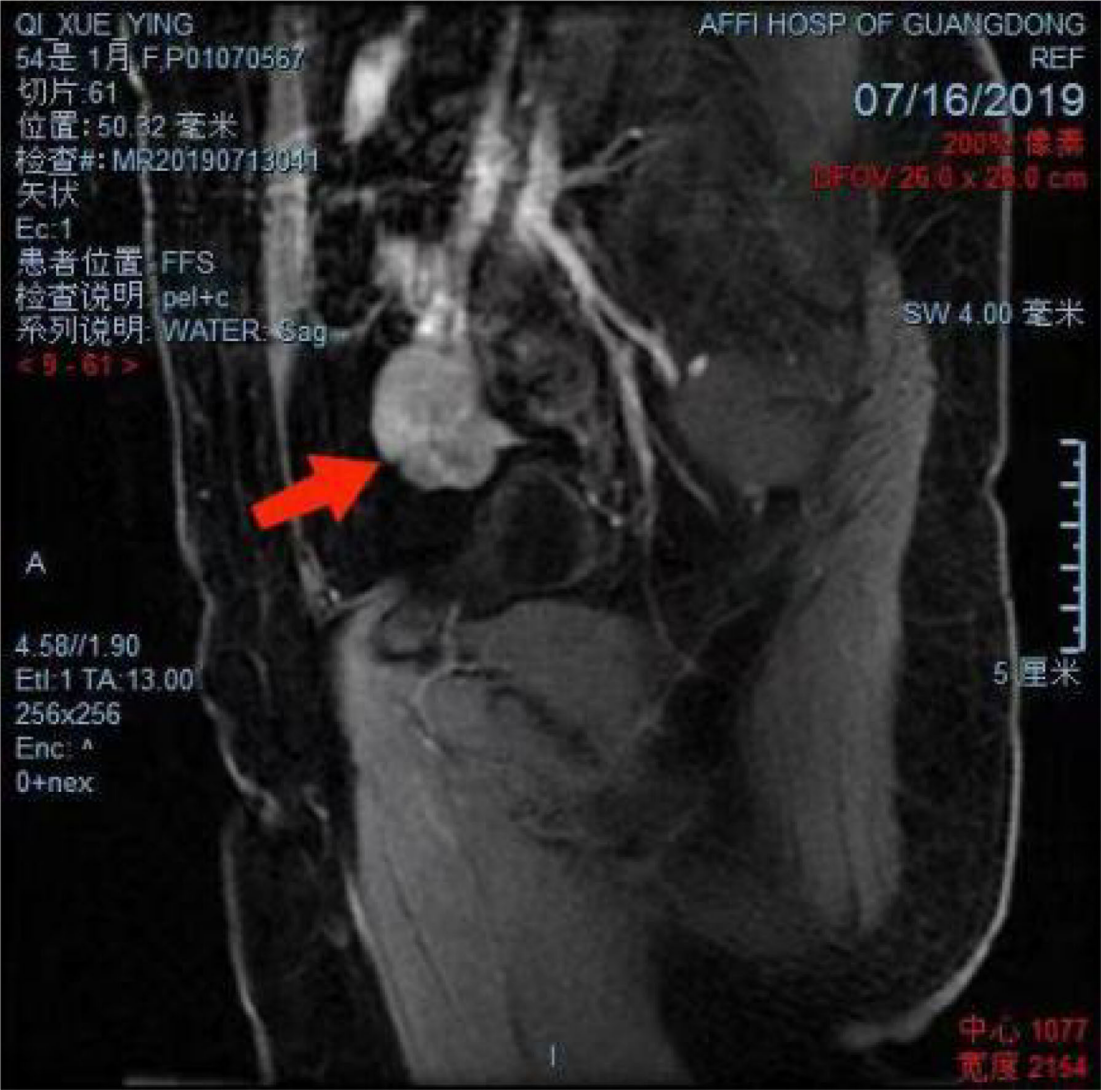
Pelvic magnetic resonance images showing a solid mass of about 2.4 cm * 3.5cm * 3.4cm on the right side of the pelvic region (arrow).
